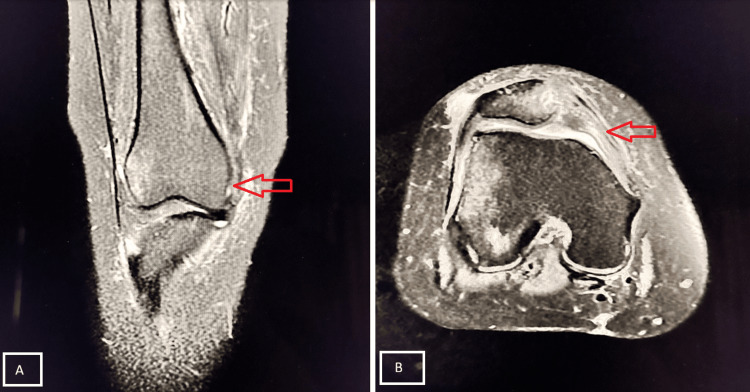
Grade II tear of the medial patellar retinaculum Coronal STIR (A) and axial PD-FS (B) MRI images demonstrate hyperintense signal and partial disruption of fibers of the medial patellar retinaculum, associated with marrow edema of the patella and mild joint effusion, consistent with Grade II injury (red arrows). PD-FS, proton density fat-suppressed; STIR, short tau inversion recovery
